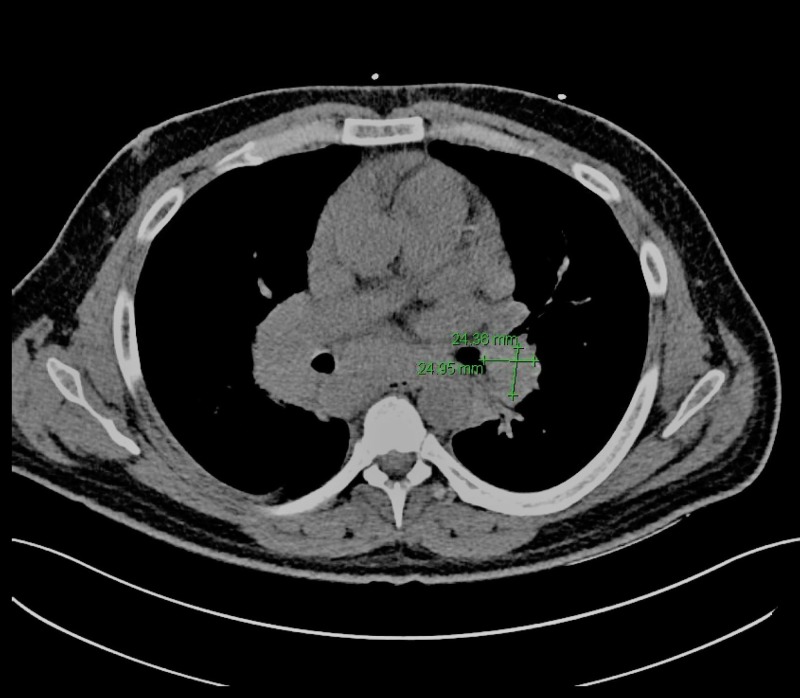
Computed tomography imaging of the chest shows enlarged left hilar lymph node measuring about 2.4 x 2.4 cm
